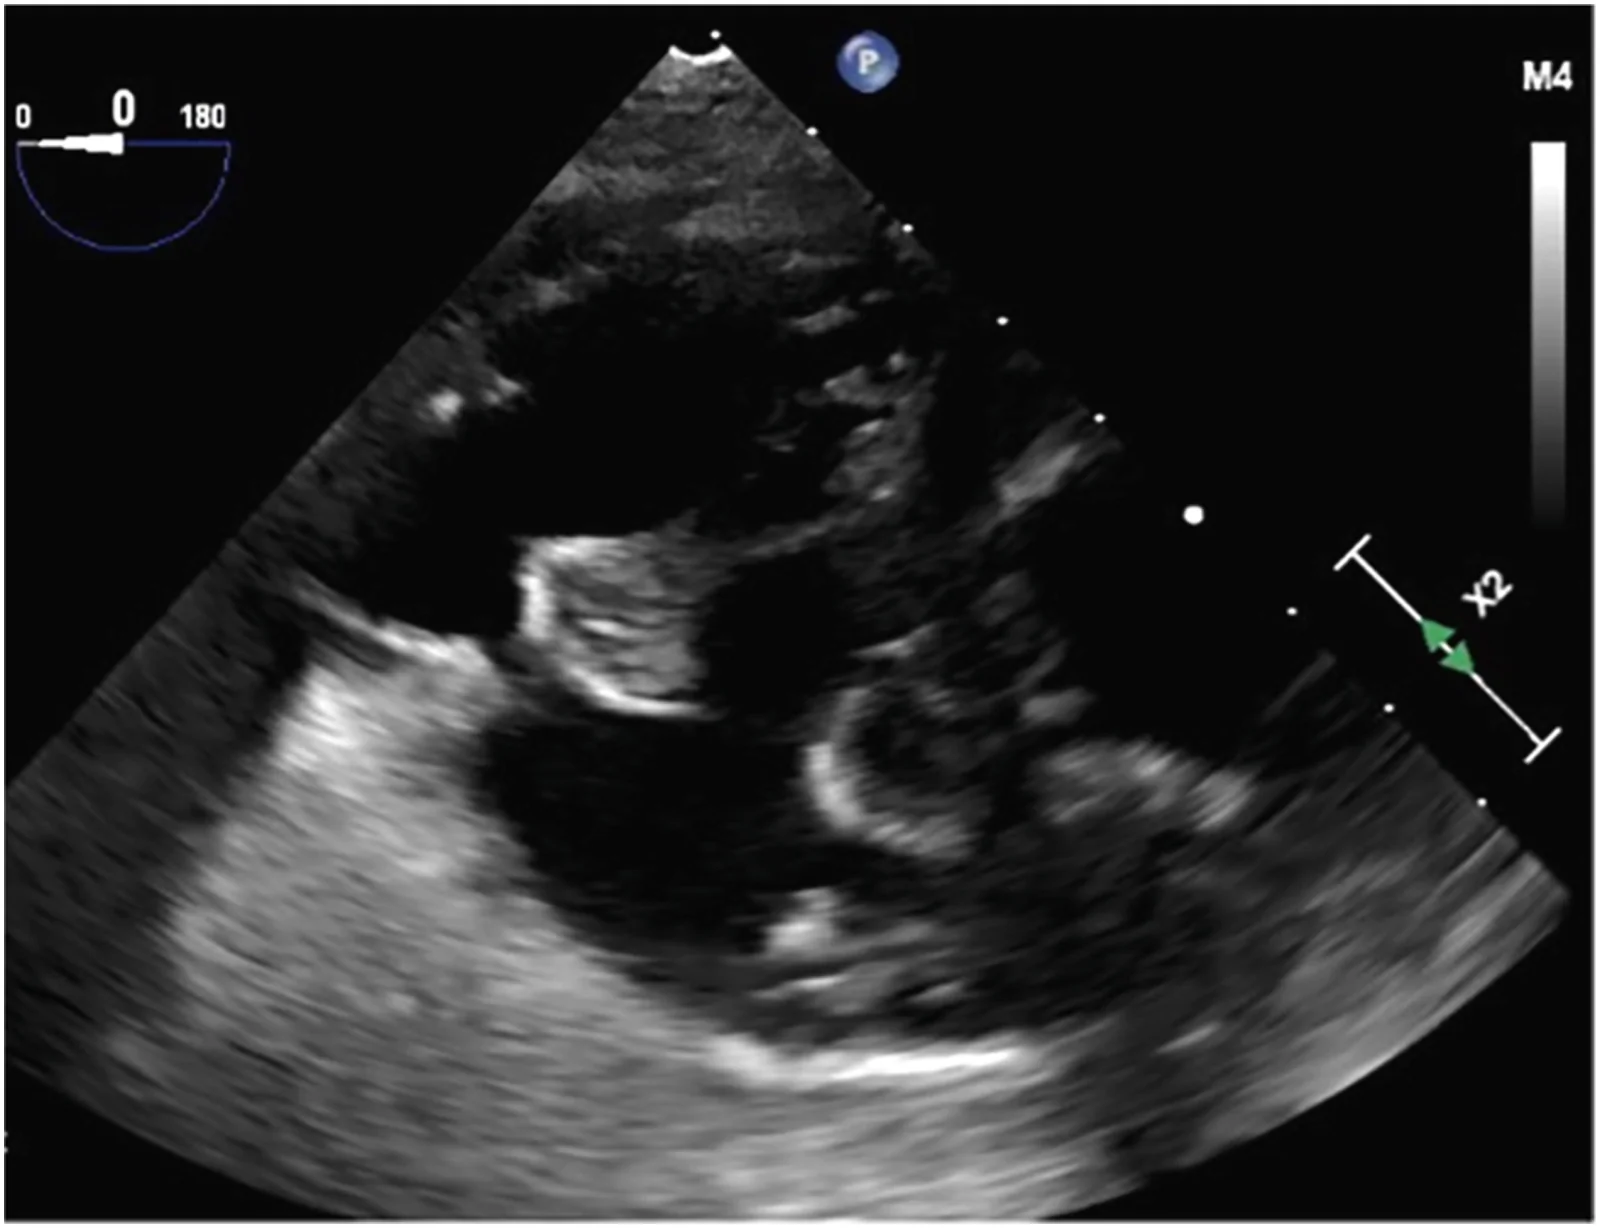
Follow-up transesophageal echocardiogram showing resolution of previous infected vegetations on the tricuspid subvalvular apparatus.
